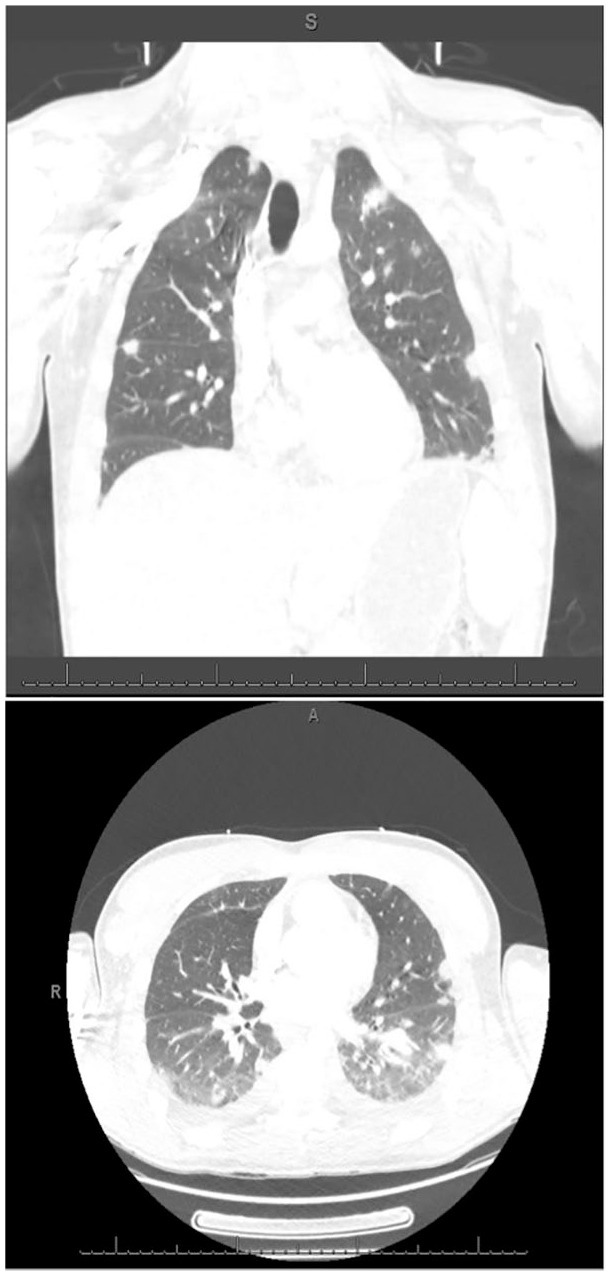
Computed tomography scan of the chest showing bilateral pulmonary nodules suggestive of septic emboli.
